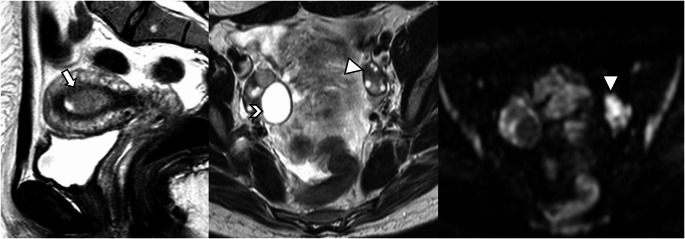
Stage IA3 grade 2 endometrioid carcinoma, no LVSI, confined to the endometrium and left ovary in a 60-year-old woman. A Sagittal T2-weighted fast spin-echo (FSE) image (TR/TE: 4500/100 ms; slice thickness: 3 mm) shows a mass confined to the endometrium without myometrial invasion (arrow). B Axial T2-weighted fast spin-echo (FSE) image (TR/TE: 4500/100 ms; slice thickness: 3 mm) and (C diffusion-weighted image (DWI) with b = 800 s/mm^2^ show a nodule in the left ovary without capsule invasion (arroweads). Cysts in the right ovary (chevron). Pathology confirmed low grade endometrial carcinoma involving both endometrium and left ovary
